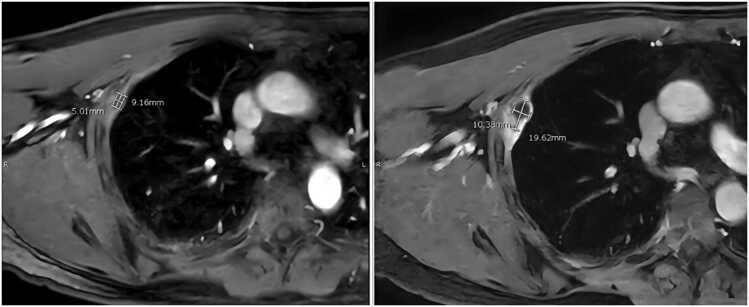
MRI after 8 weeks of treatment revealed significant regression of the pleural hydatid cyst, with the lesion size decreasing from 12 × 10 mm to 7 × 4 mm and resolution of surrounding inflammation.
